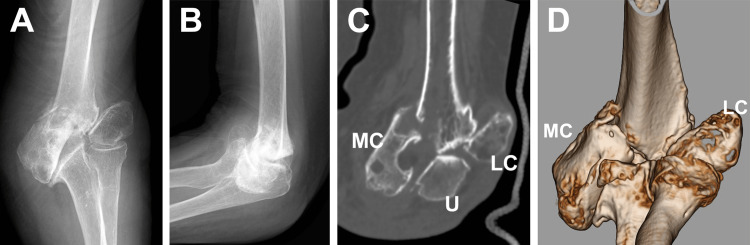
Preoperative radiographic images. The plain radiographs (A: anteroposterior image, B: lateral image) and CT images (C: coronal image, D: three-dimensional image) represent the medial condyle fracture in the presence of fishtail deformity and lateral condyle nonunion. MC, medial condyle; LC, lateral condyle; U, ulna
